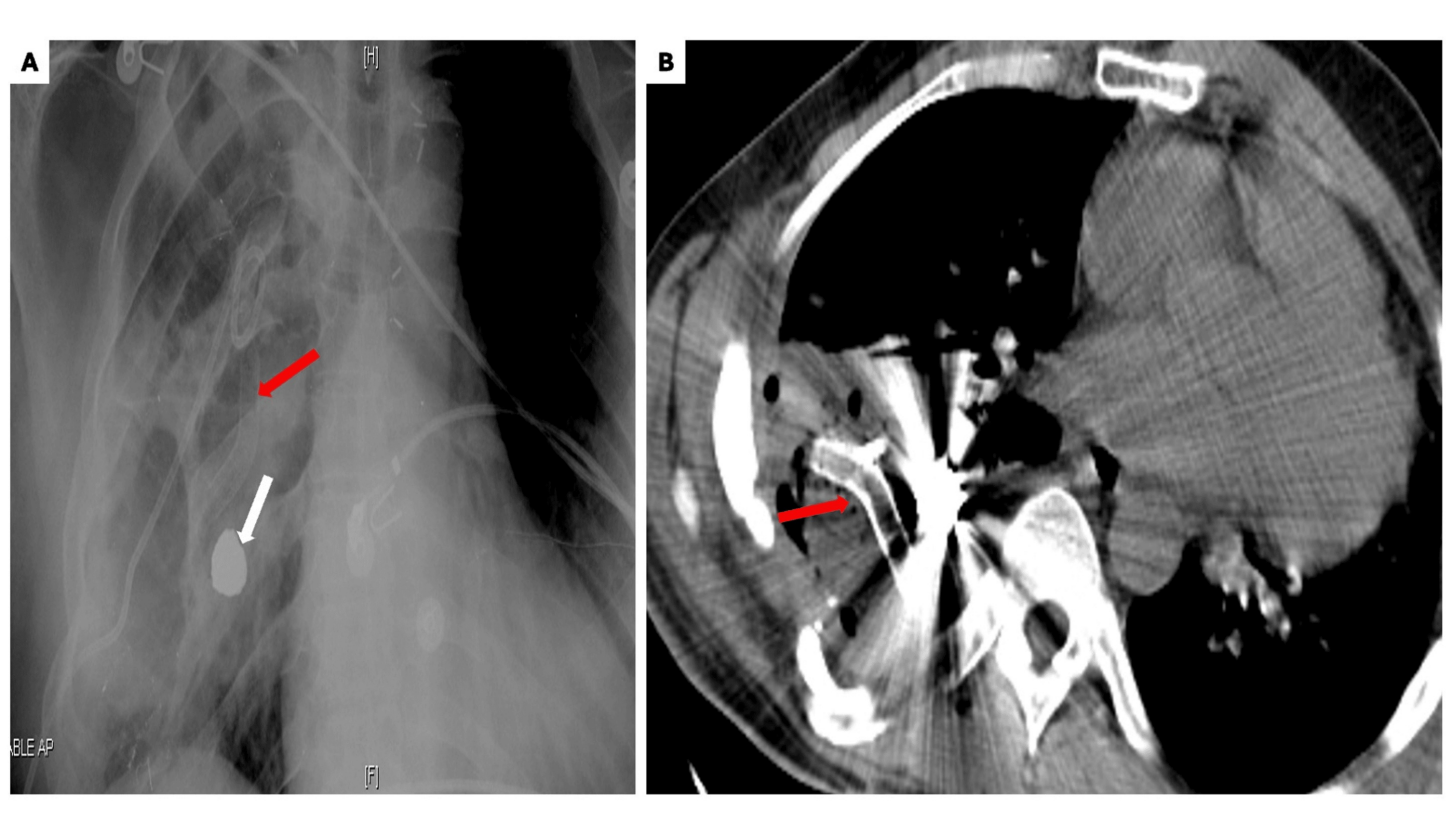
Imaging studies depicting stent migration (A) Radiograph and (B) Computed tomography demonstrating migration of the stent (red arrow) and ballistic fragment (white arrow) into the right thoracic cavity.
